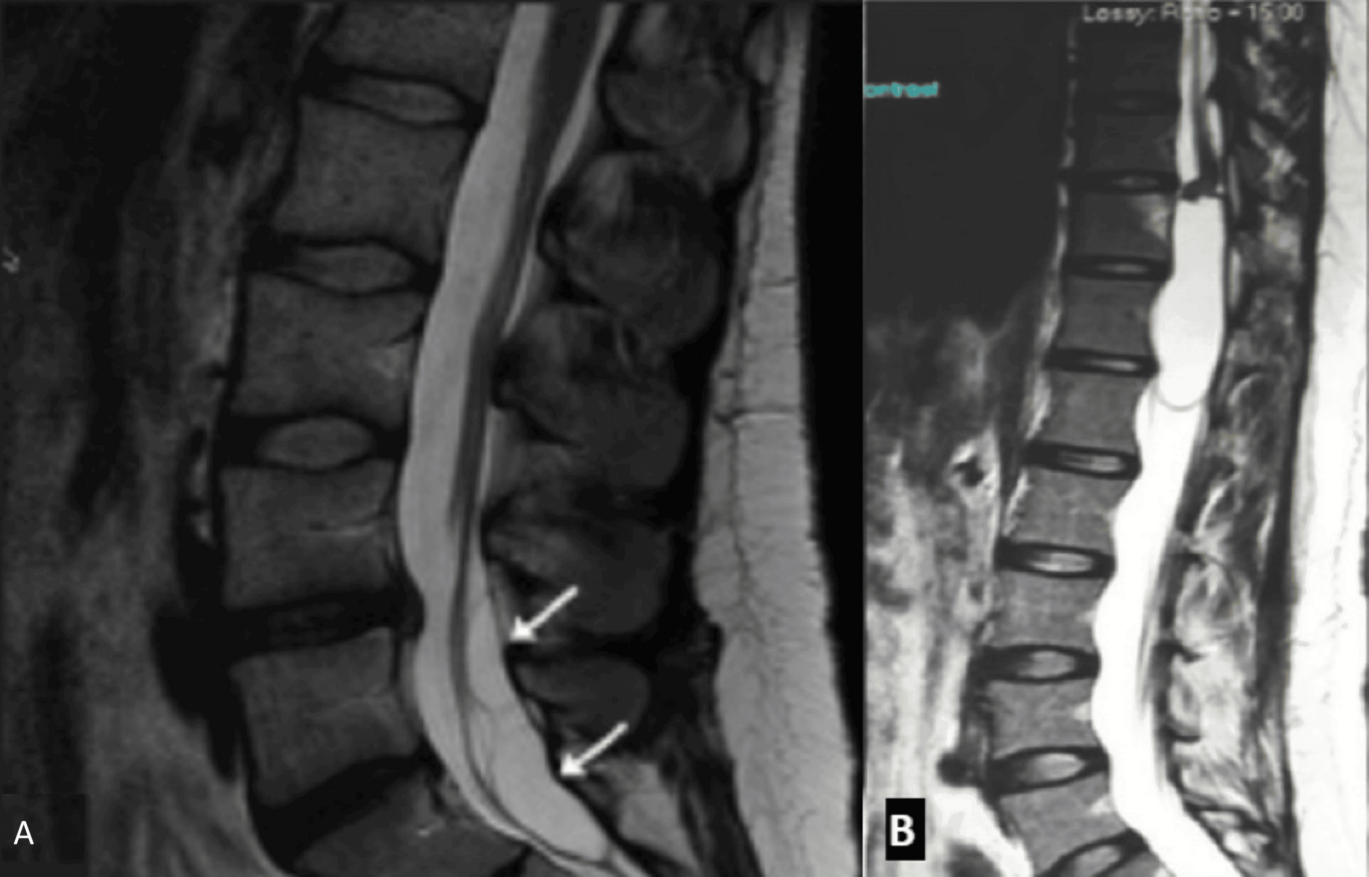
Post-epidural spinal arachnoid cyst. Image A is a sagittal axial T2-weighted MRI of the lumbrosacral spine showing two well-defined, loculated, intradural arachnoid cysts (arrows) in a 36-year-old female patient [9]. Image B is a sagittal axial T2-weighted MRI of the lumbrosacral spine showing an intradural extramedullary cyst in spinal levels T11 through L1 in a 43-year-old female patient [10].
